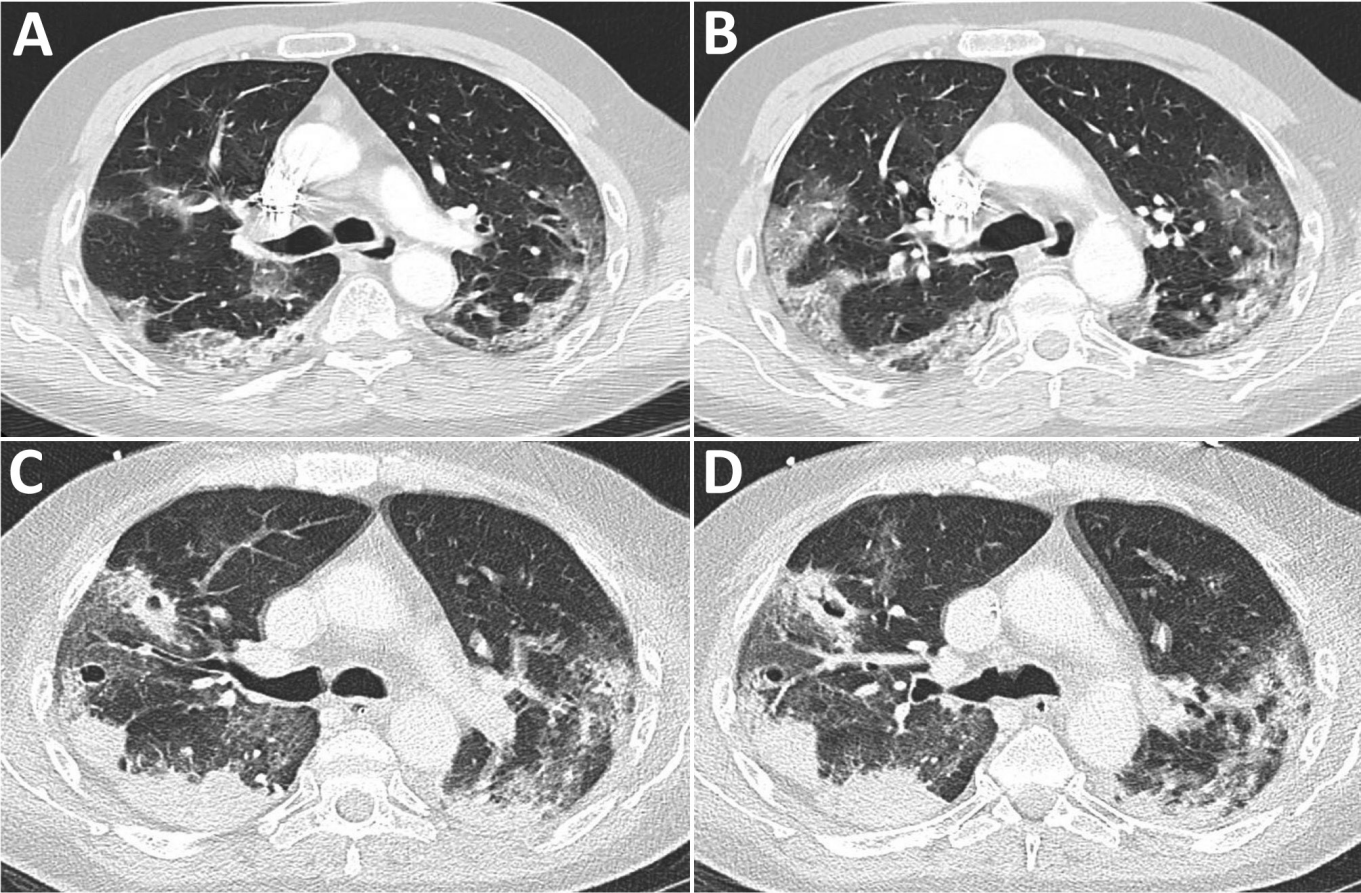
Computed tomography scans of patient with *Bordetella hinzii* pneumonia and severe respiratory syndrome coronavirus 2 infection. A, B) Scan at admission showing bilateral evidence of extensive areas of mainly crazy paving patterns with some posterior consolidations. C, D) Scan at day 25 showing marked increased extent of consolidation.
